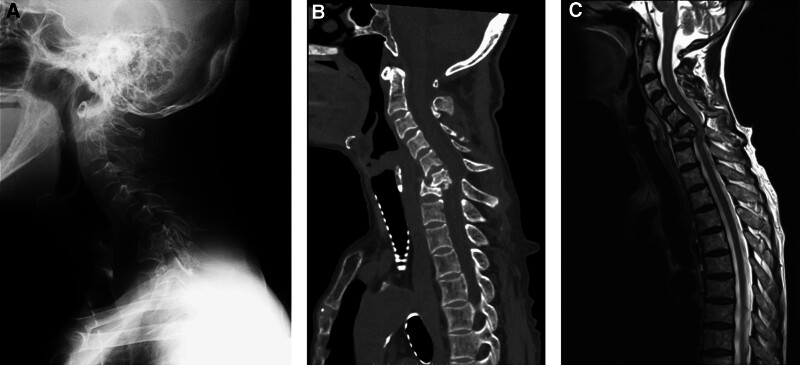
A 61-year-old female with chronic kidney disease (CKD) presented with destructive spondyloarthropathy (DSA) on C6 and C7. Lateral views of (A) the X-ray, (B) computed tomography (CT), and (C) Magnetic resonance imaging (MRI) T2 weighted image (T2WI).
